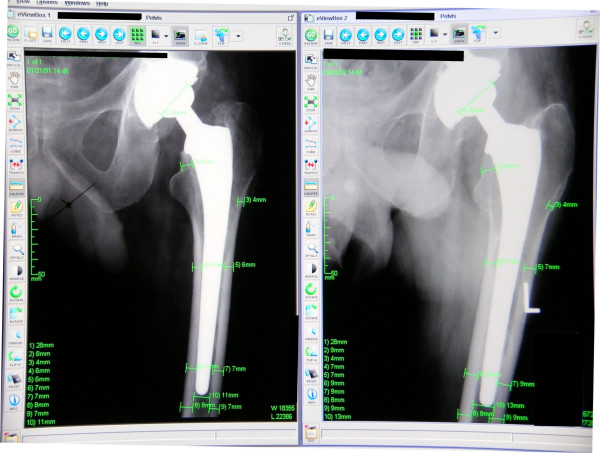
Image taken from the picture archiving and calibration system, demonstrating the areas measured on the immediate postoperative (left) and most recent anteroposterior radiographs (right), with magnification calibrated from the known diameter of the modular femoral head component.
